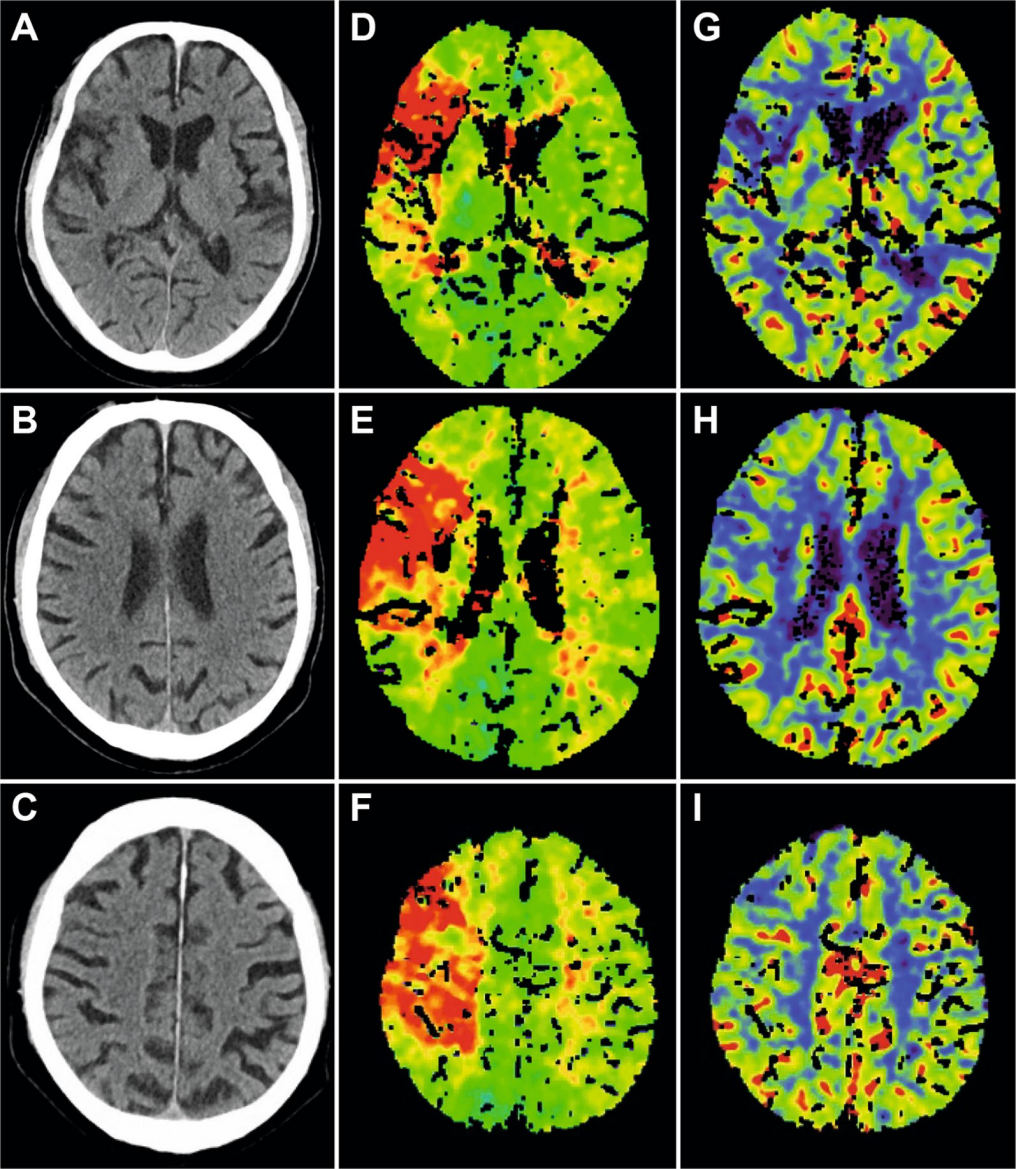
Initial imaging of patient 1. Non-contrast CT images (A–C) and CT-perfusion imaging including time to peak (TTP; D–F) and cerebral blood volume maps (CBV; G–I) on admission are provided. On initial imaging, minimal early ischemic changes were present (A–C), contrary to the large mismatch (TTP/CBV) detected by perfusion imaging (D–I)
